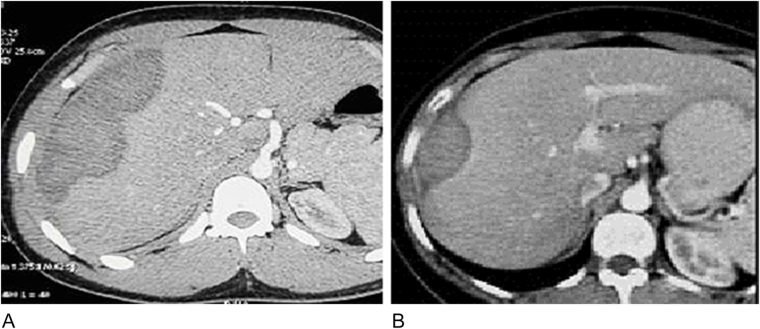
(A) Follow-up contrast-enhanced computed tomography (CECT) of the abdomen and pelvis (early arterial phase) performed 4 months after presentation (10 January 2025), demonstrating maximal resolution of the subcapsular hepatic hematoma with restoration of normal hepatic contour. (B) Follow-up contrast-enhanced computed tomography (CECT) of the abdomen and pelvis (late arterial phase) performed 5 months after presentation (6 February 2025), demonstrating near-complete resolution of the subcapsular hepatic hematoma with restoration of normal hepatic contour.
